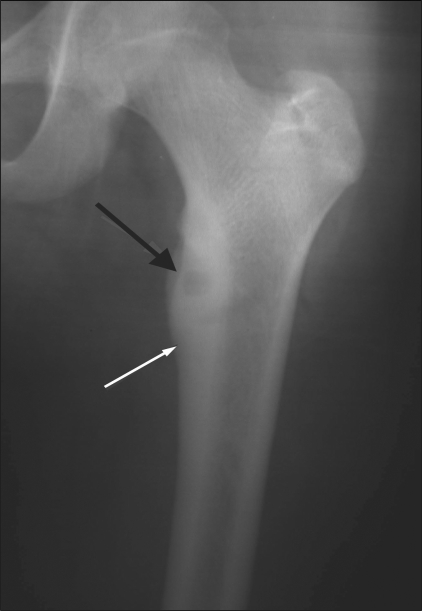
Osteoid osteoma. Plain radiograph shows a typical metadiaphyseal cortical osteoid osteoma involving the upper end of the femur. The nidus is well seen (black arrow) along with the surrounding cortical thickening (white arrow)
